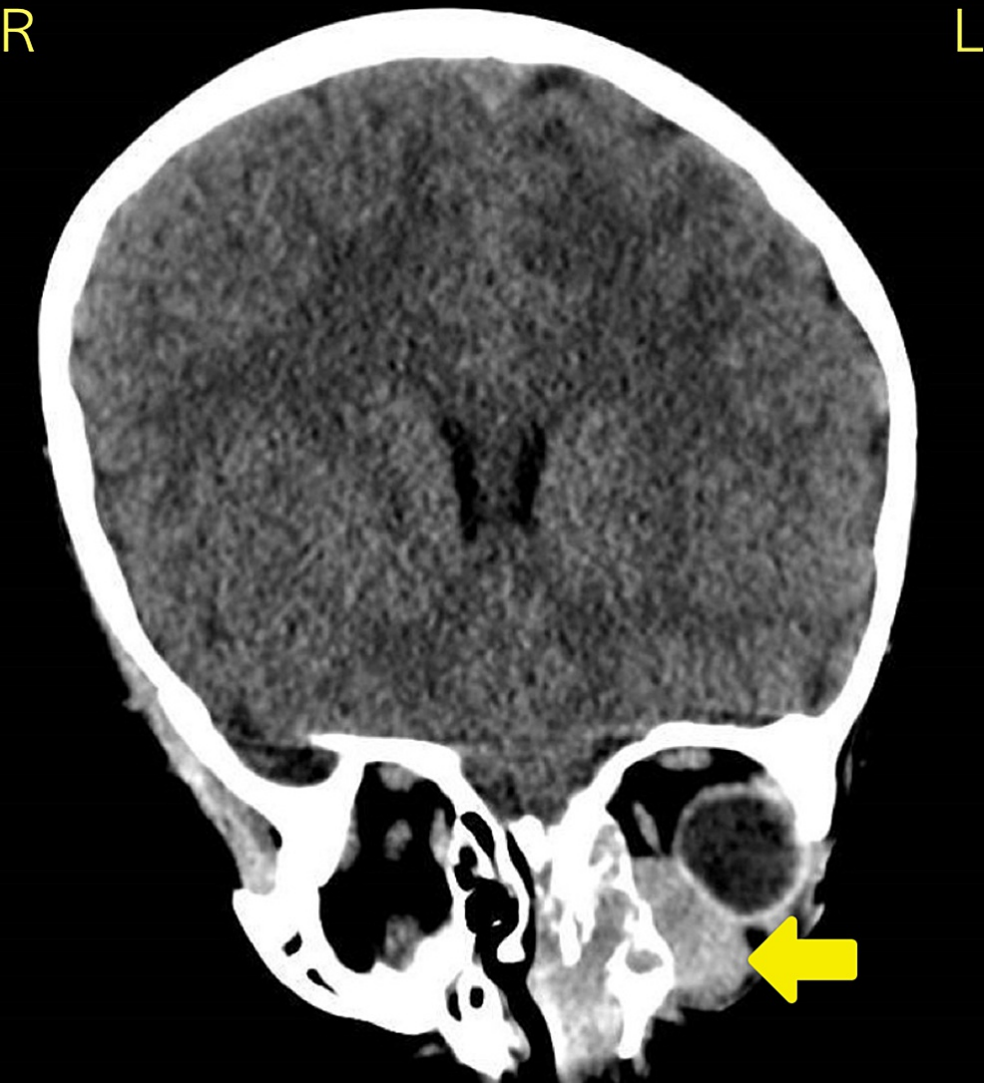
Coronal CT brain and paranasal sinuses for case 3 revealed a left-sided orbital mass (arrow) occupying the inferonasal aspect of the orbit. There is local invasion through the lamina papyracea of the left ethmoid air cells, nasal cavity, and mild displacement of the nasal septum.
